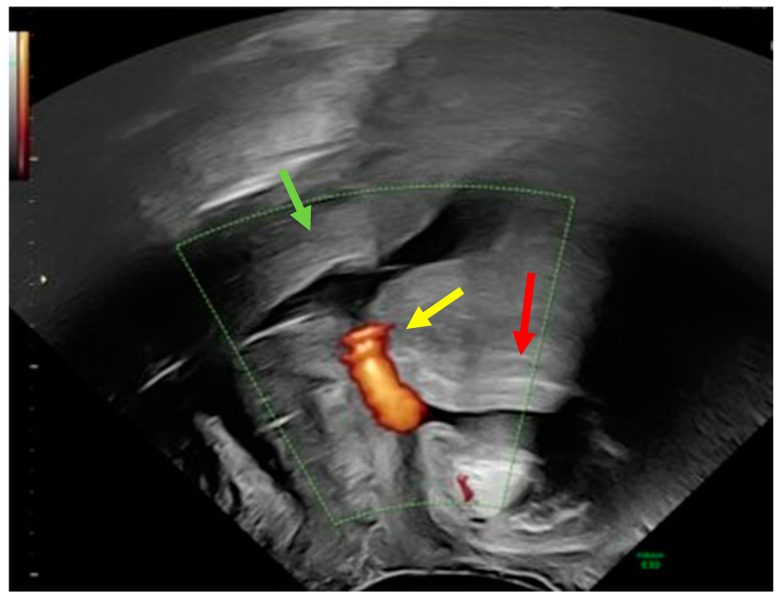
Transvaginal ultrasound using power Doppler demonstrating communication (yellow arrow) between the uterine cervix (green arrow) and bladder (red arrow).
